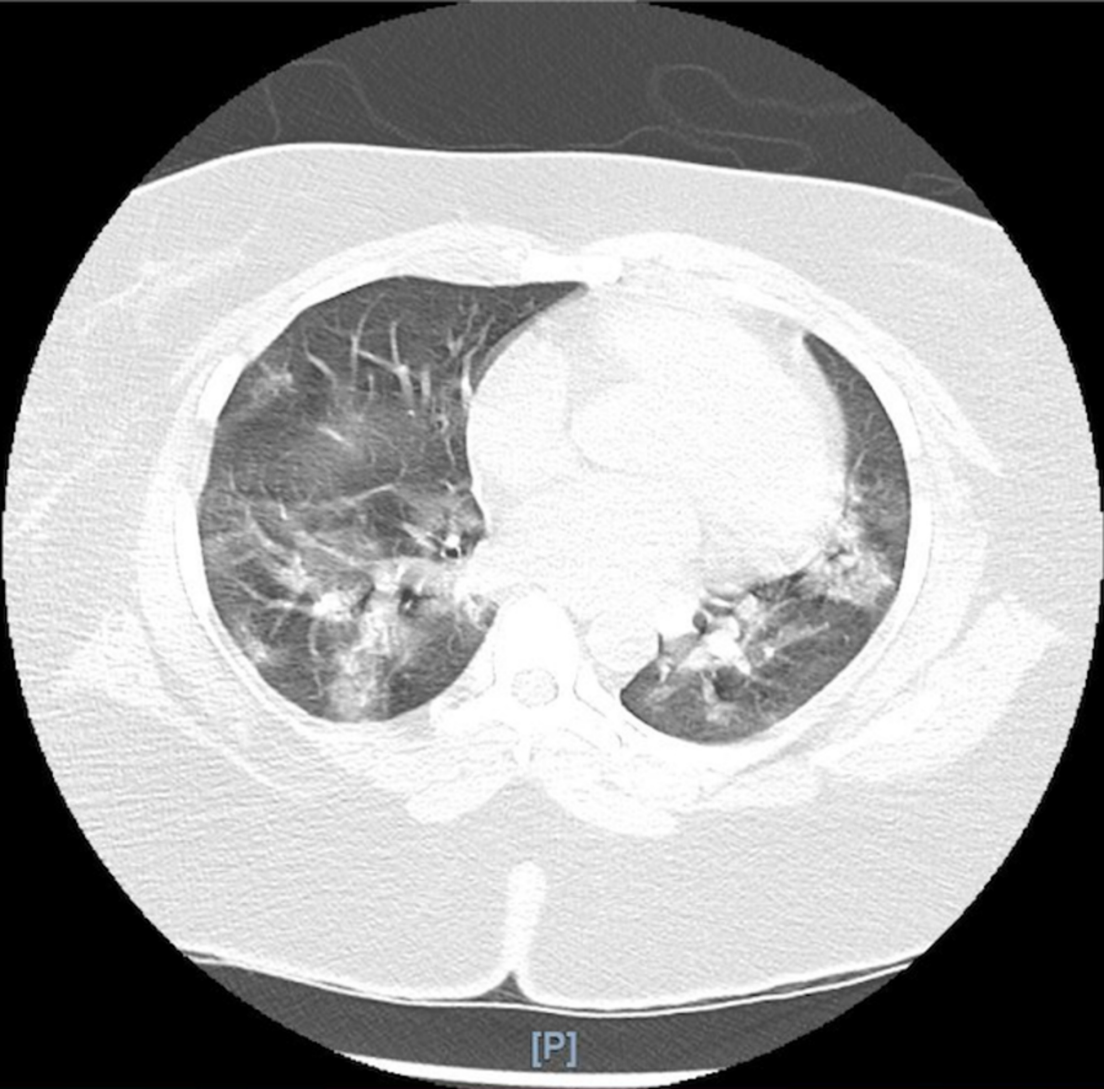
Chest CT without contrast demonstrating patchy, bilateral ground-glass opacifications.
